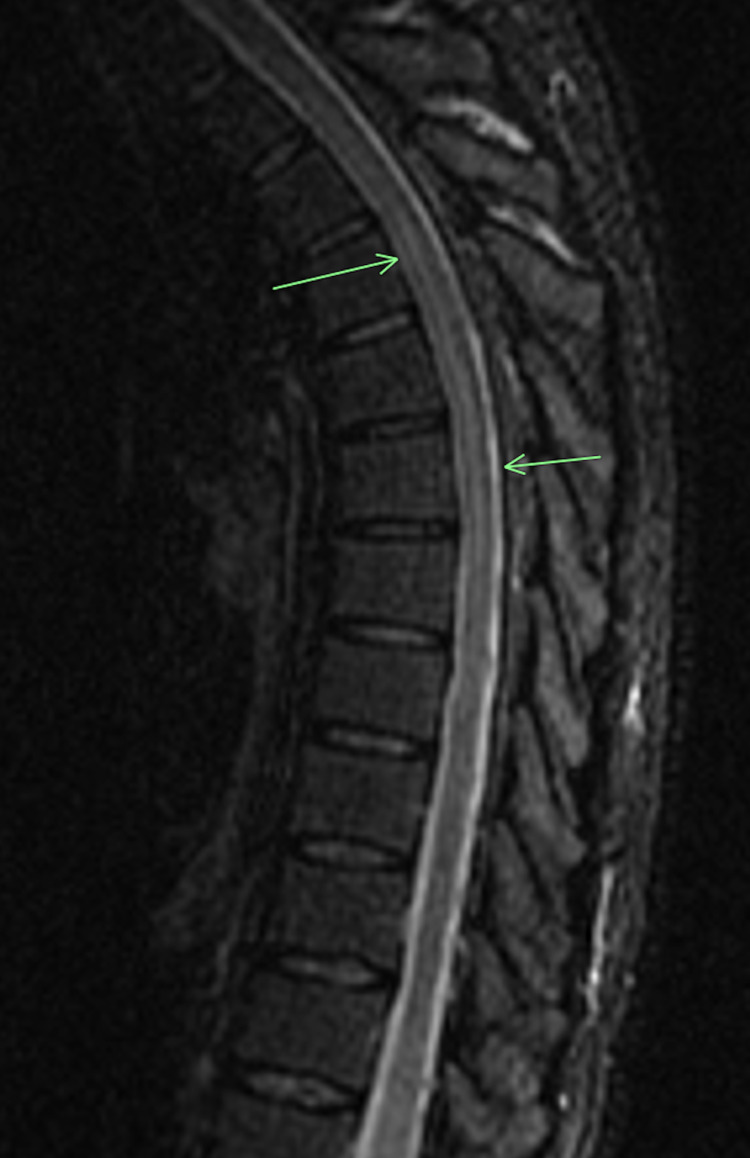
Short-tau inversion recovery MRI of the thoracic spine revealing mild intramedullary signal abnormality at T3-T4. MRI: magnetic resonance imaging
